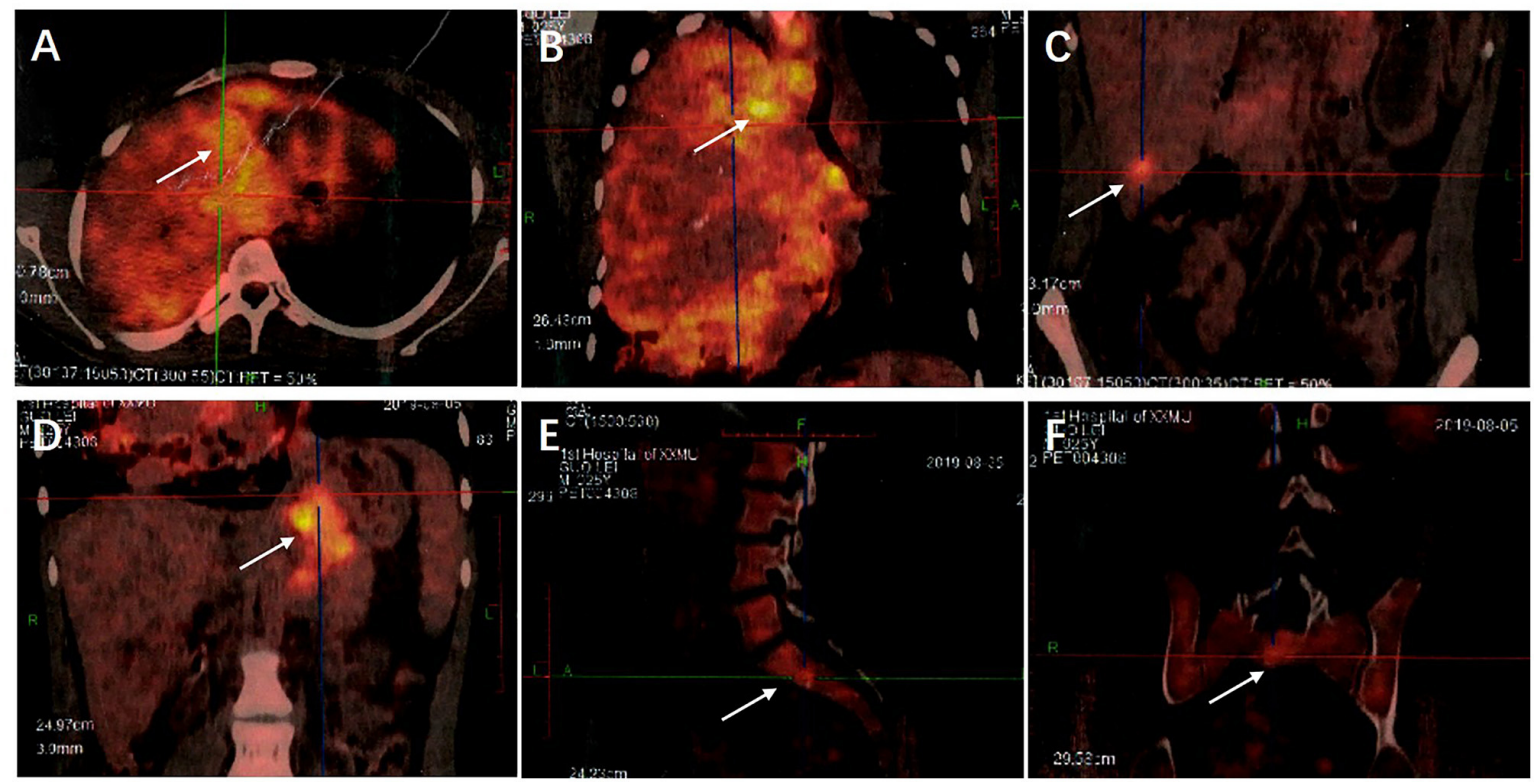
(A) The 18F-FDG PET/CT examination showed a huge irregular mass in the right lung with uneven density and increased FDG uptake. The SUVmax was 8.21. (B) Multiple enlarged lymph nodes were seen in the 2R, 2L, 4R, 4L, 5, 7, 8, and 10R areas of the mediastinum, their FDG intake had unevenly increased and the SUVmax was 10.39. (C) Round low-density nodules were present in the lateral segment of the left lobe of the liver and the posterior lower segment of the right lobe of the liver, respectively. Round low-density nodules were also present in the left and right lobes of the liver, respectively, with unclear borders and increased FDG uptake. The SUVmax was 4.48. (D) Multiple enlarged lymph nodes were present in the abdominal cavity, there was an increase in FDG uptake and the SUVmax was 6.79. (E,F) Low-density lesions were seen in the lumbar 1 and sacral 1 vertebral bodies, FDG uptake had increased and the SUVmax was 4.58. Examination through 18F-FDG PET/CT showed a malignant tumor in the right lung with multiple metastases in the hilar and mediastinal lymph nodes, several bones, liver, and intra-abdominal lymph nodes.
